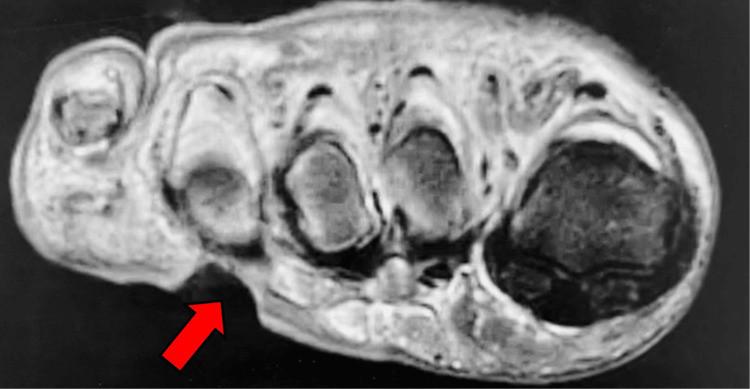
Axial MRI Without Contrast of the Right Foot Lower axial plane of MRI shows ulceration of the plantar soft tissues near the fourth metatarsal head (red arrow).
